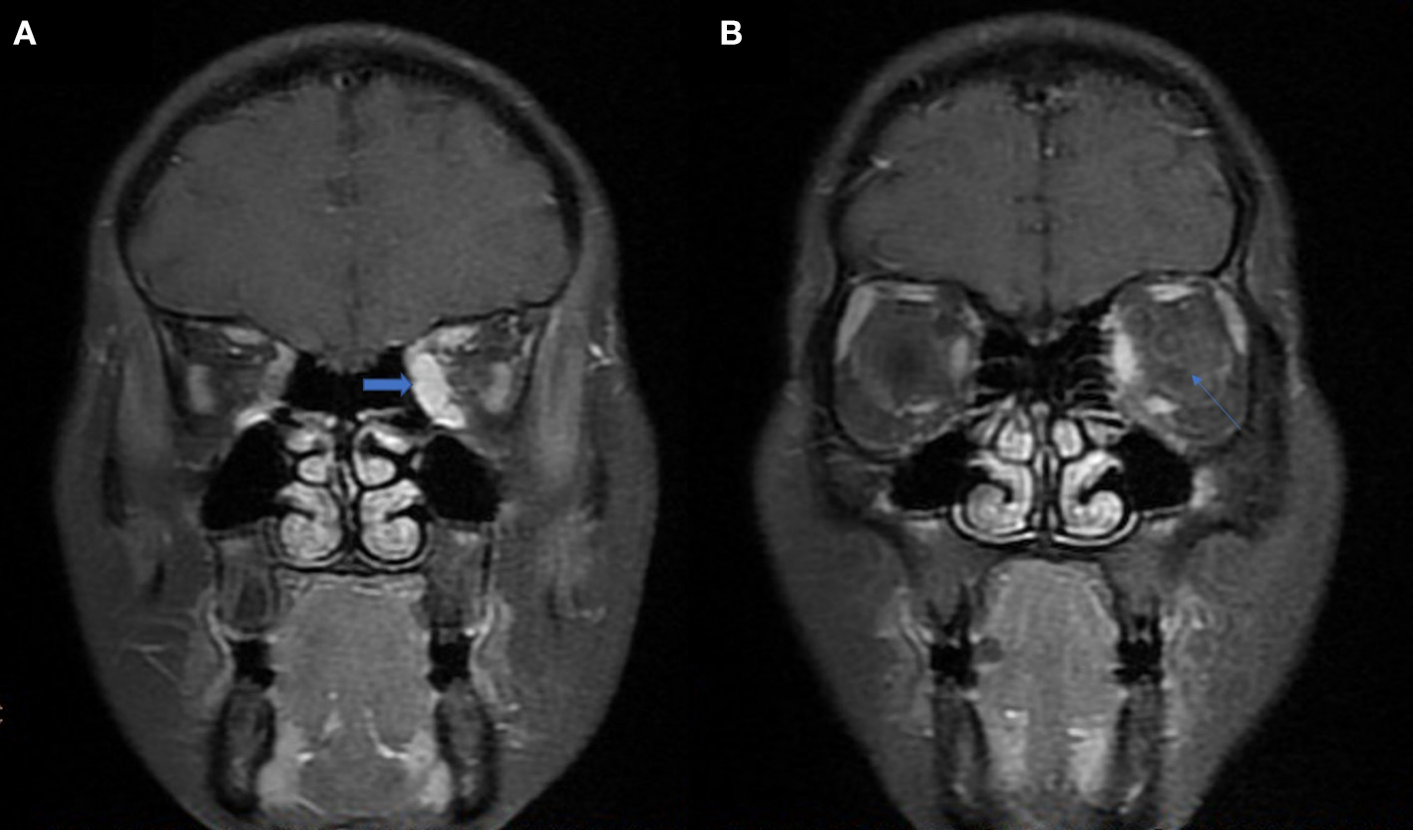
MRI findings of OPN patients with Graves' disease. (A) Coronal contrast enhanced T1-weighted MRI showing extraocular muscle belly enlargement (arrow). (B) Coronal contrast enhanced T1-weighted MRI showing thickening and enhancement of the left optic nerve sheath (arrow).
